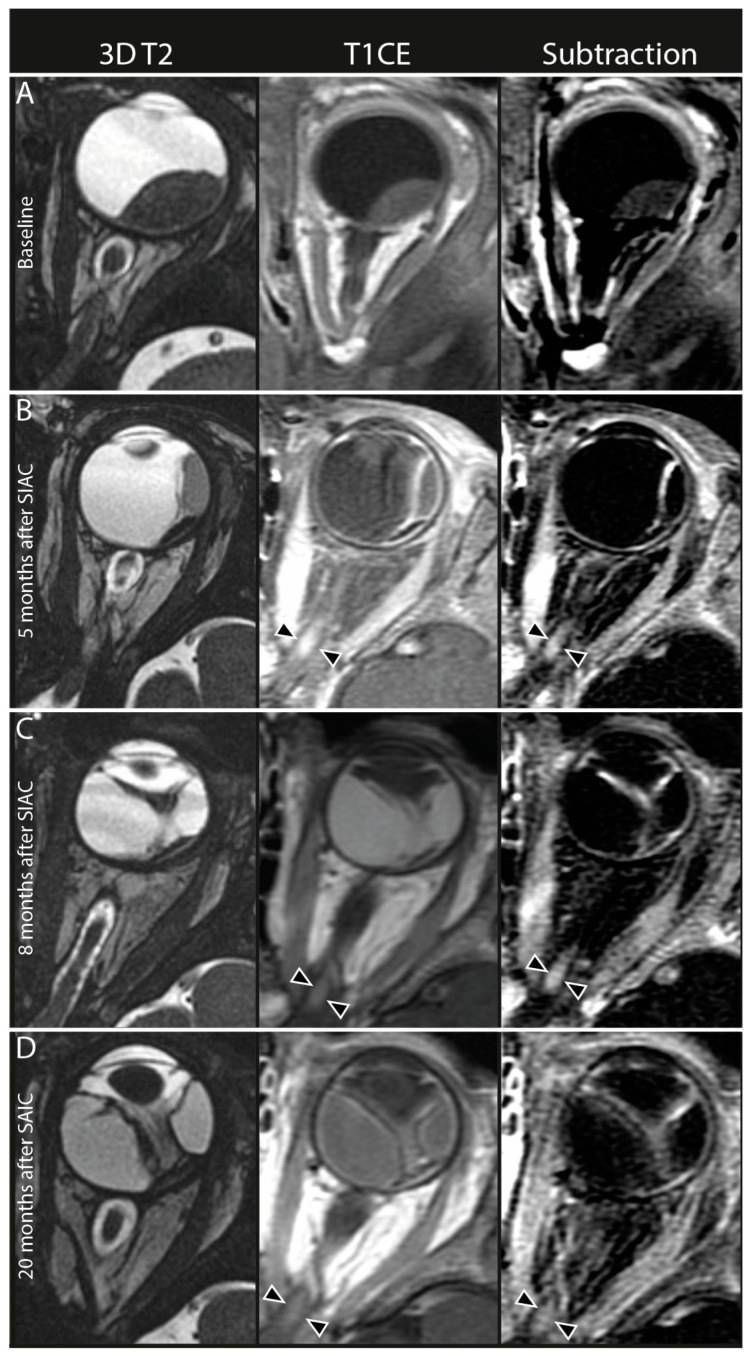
Persistent optic nerve enhancement after selective intra-arterial chemotherapy (SIAC). In the left column, axial 3D T2-weighted MR images (3D T2), middle column axial contrast-enhanced T1-weighted MR images (T1CE), and in the right column axial subtraction images from contrast-enhanced T1-weighted MR images minus native T1-weighted MR images (subtraction). (A) Pretreatment MRI scan showed no optic nerve enhancement in any part of the optic nerve. (B) First follow-up MRI 5 months after the last SIAC cycle (a total of three cycles of combined 10 mg melphalan [3 mg for the first and second cycle, and 4 mg for the last cycle]. The procedure was conducted through the carotid artery with the catheter tip in the ostium of the ophthalmic artery with no wedging or complications. Enhancement of the proximal part of the optic nerve (black arrowheads). Persistent enhancement of the optic nerve 8 (C) and 20 (D) months after the last SIAC cycle (black arrowheads).
